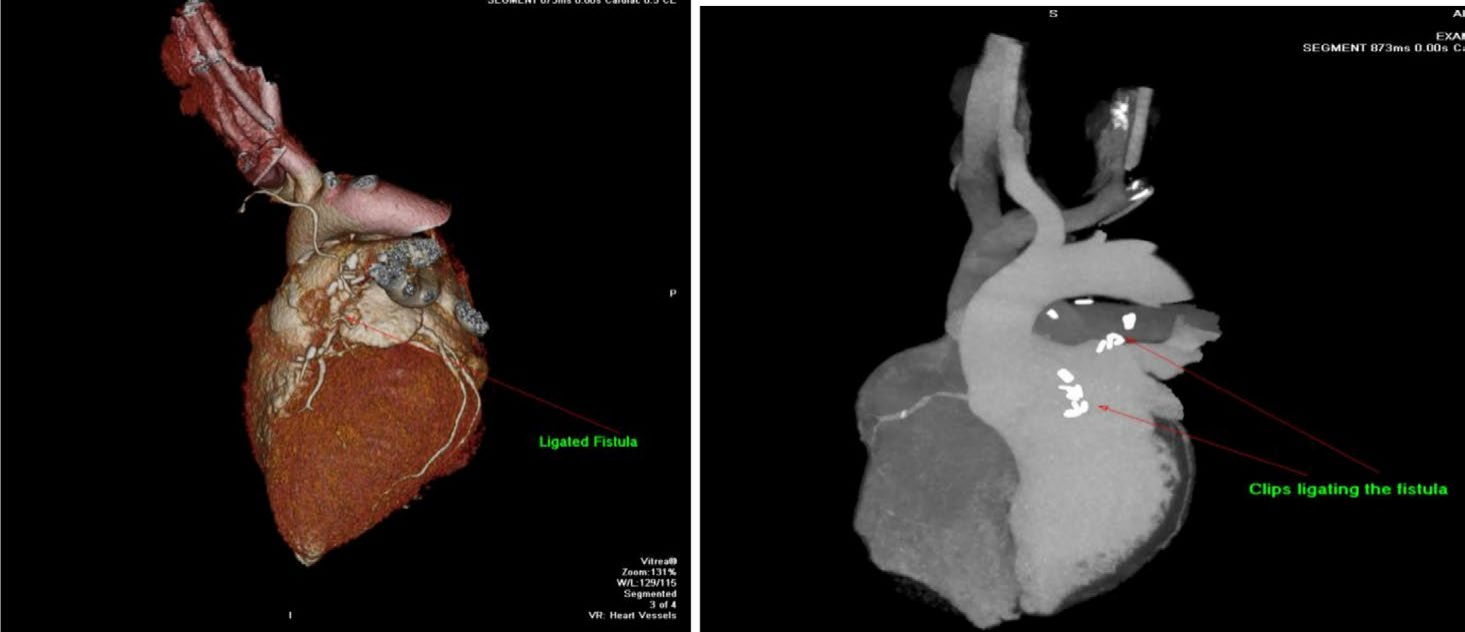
Postoperative CT coronary angiogram showing ligated fistula.
